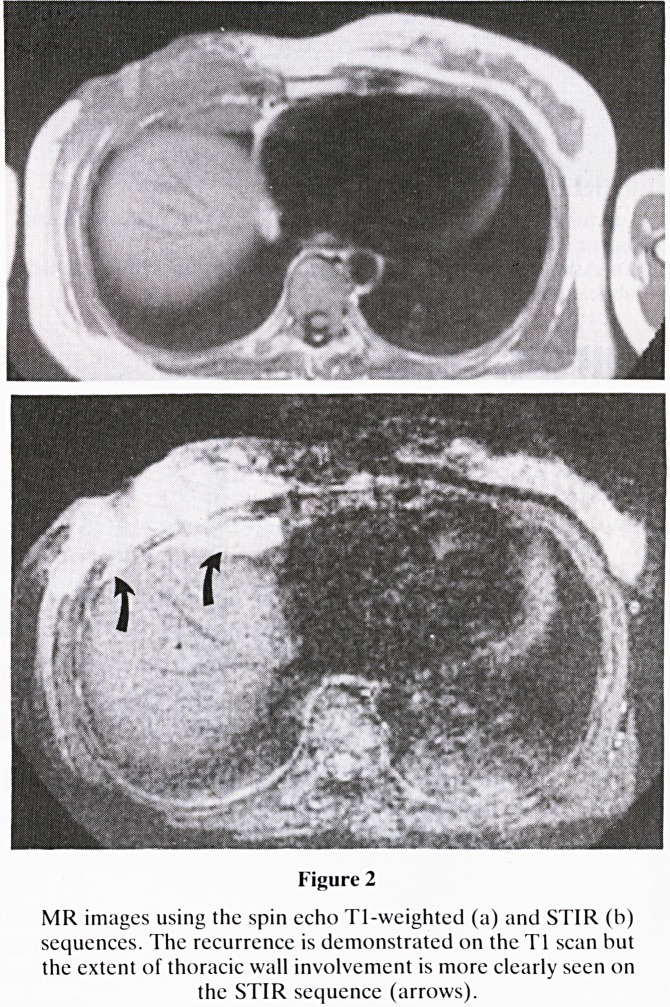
# Magnetic Resonance Imaging and Carcinoma of the Breast
